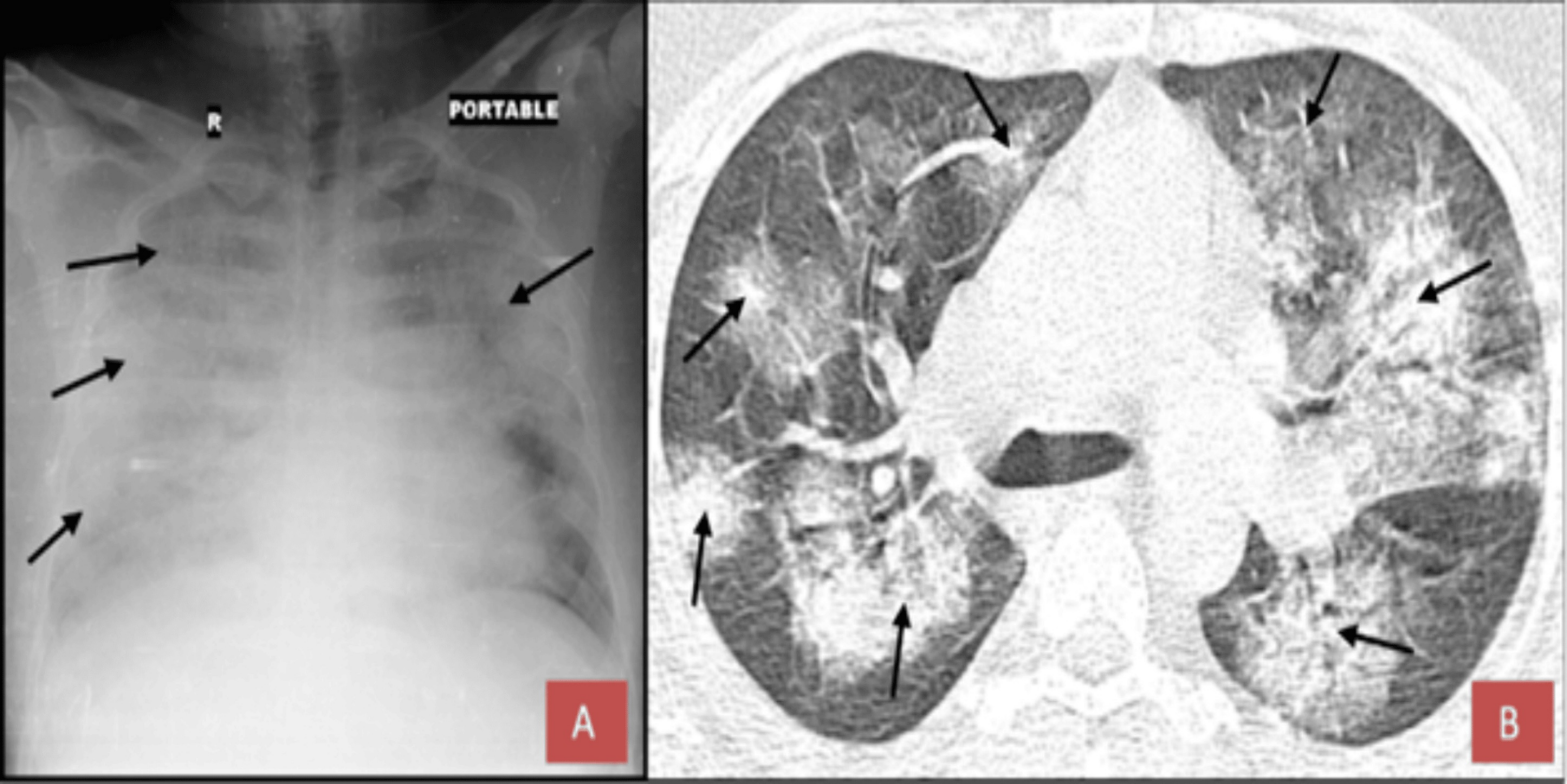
Findings X-ray (A) and CT scan (B) were performed, revealing a CT severity score of 23/25 upon admission. Arrows indicate regions of pneumonitis accompanied by pulmonary edema, leading to a complete whiteout of the lung.
